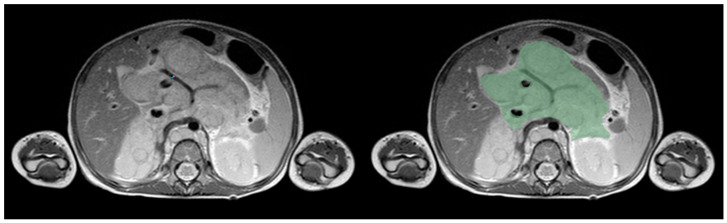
Example of axial MRI slice with tumor segmentation. Example of manual tumor segmentation on axial T2-weighted MRI. Primary tumor was segmented slice by slice to generate three-dimensional volume of interest. Abbreviations: MRI, magnetic resonance imaging.
